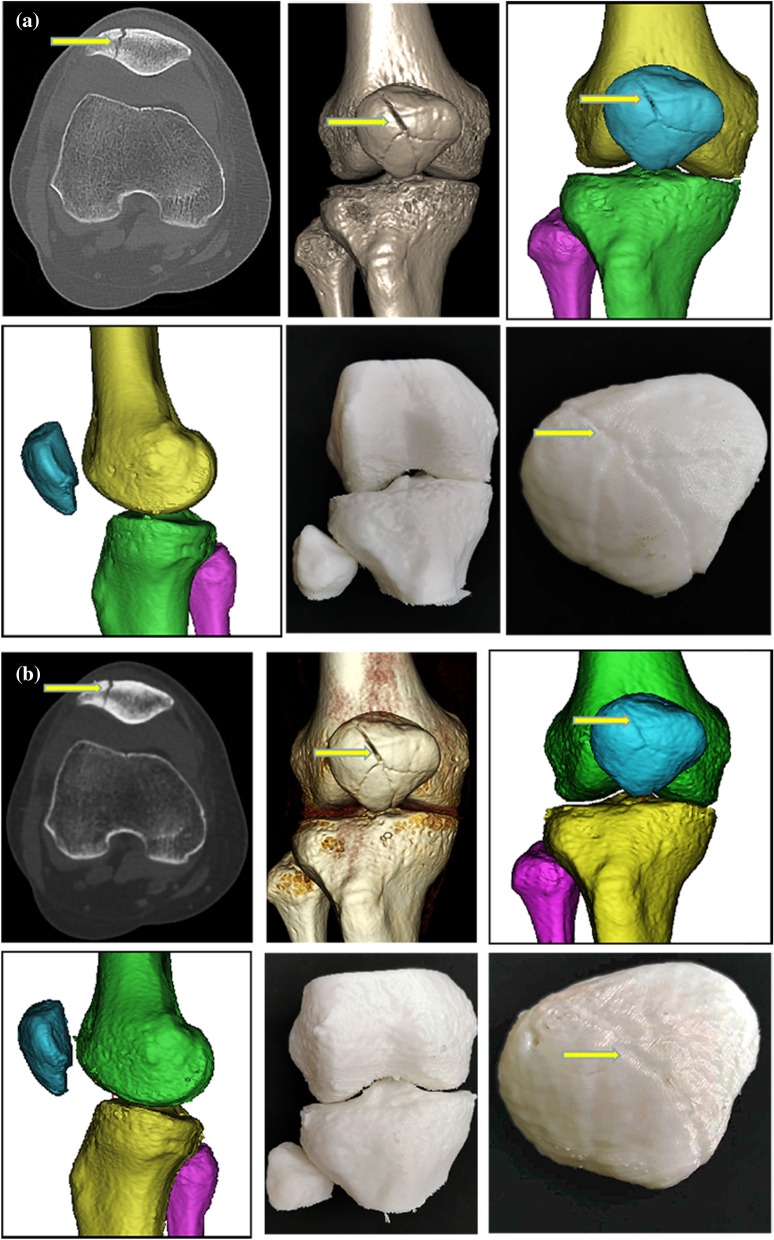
Representative case 3. A 47-year-old woman with undisplaced patella fracture underwent two scans (at the first visit and 10 days later). The standard-dose (a) and ultra-low-dose (b) protocols were used to generate 3-D printing models from 3D CT data. a-1 and b-1 CT axial images with quality score of 3 points in a and 2 points in b. The fracture lines (yellow arrows) are identifiable in both scans, meeting the needs for clinical diagnosis and treatment. a-2 and b-2 Maximum intensity projection images. a-3–4 and b-3–4 3D reconstruction of Mimics research medicine images. a-5–6 and b-5–6 3DP images with score of 3 points in a and 2 points in b. The surface of the bone models is regular and flat, which is suitable for preoperative evaluation
